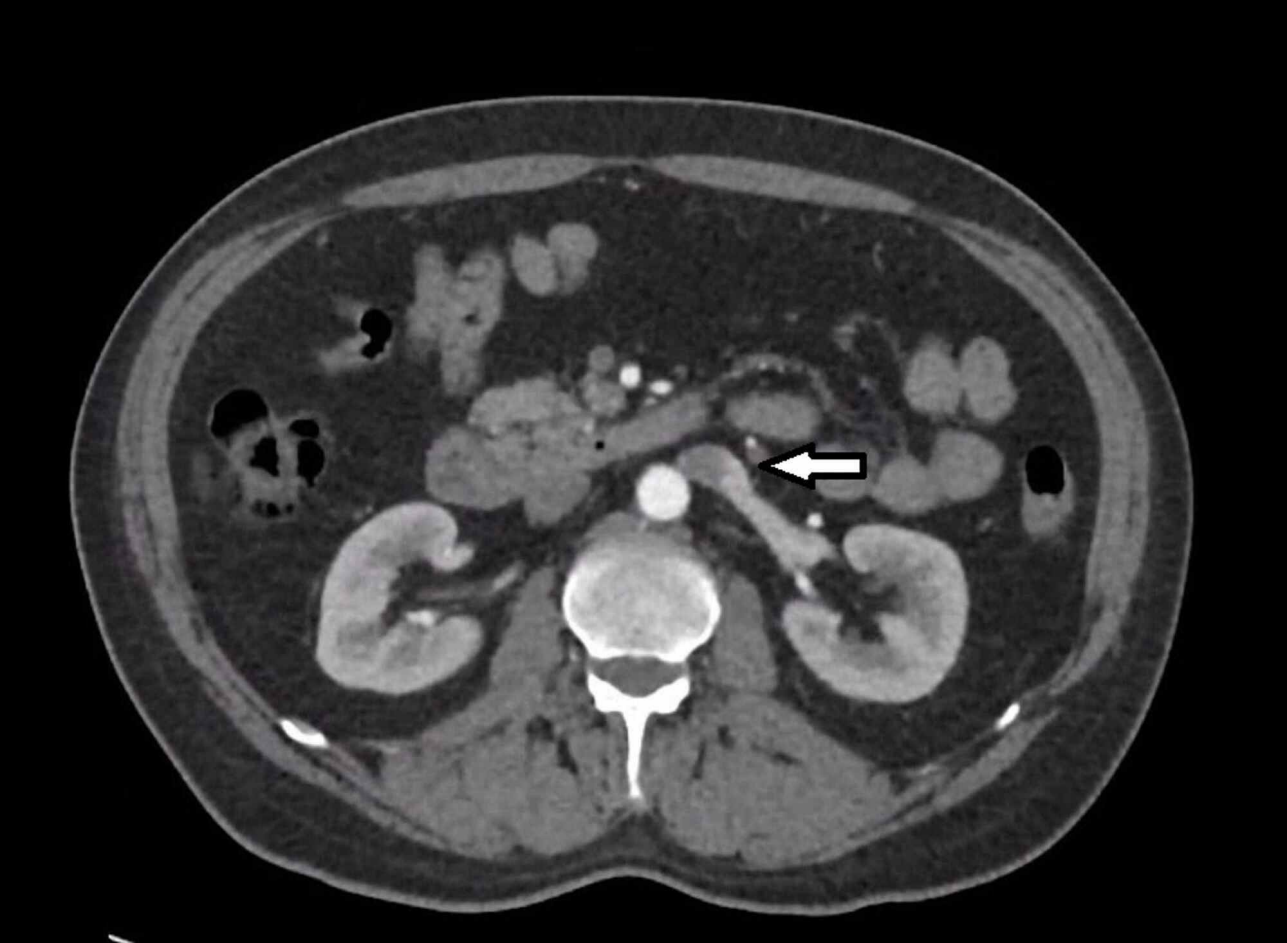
CT abdomen (axial): Bilateral vena cava on each side of the abdomen
